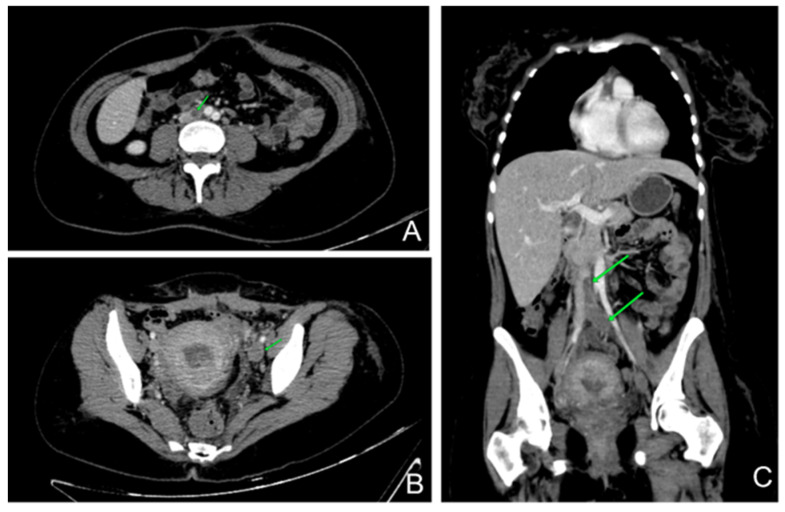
Contrast-enhanced thorax abdominal and pelvis CT: (A)—axial abdominal CT shows thrombus in inferior vena cava; (B)—axial pelvis CT reveals the extension of the thrombus in the left external iliac vein; (C)—coronal CT encompasses the length of the thrombus.
